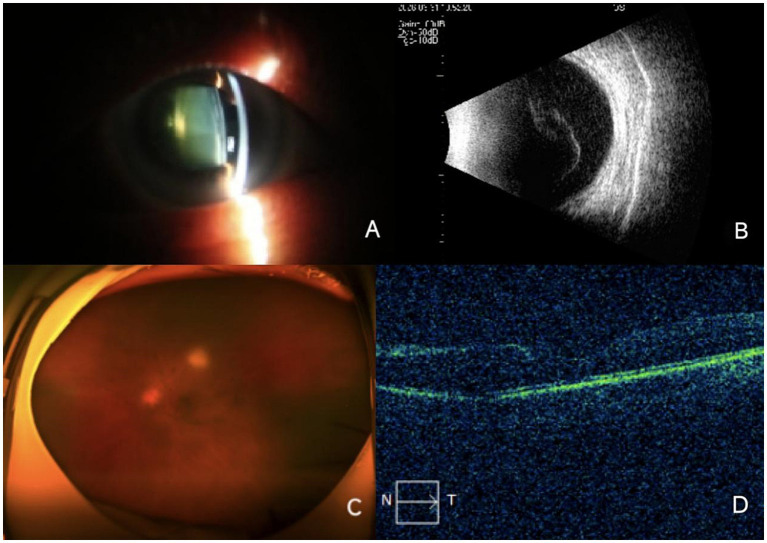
Pre-treatment images of the affected left eye. (A) Anterior segment photography showed marked lens opacity. (B) Pretreatment ocular B-scan ultrasonography revealed scattered vitreous opacities within the vitreous cavity. (C) Scanning laser ophthalmoscopy (SLO) showed inflammatory vitreous haze in the left eye, with an indistinct yellowish-white triangular lesion approximately 1 disc diameter in size at the superior vascular arcade; the retinal image quality was poor. (D) OCT showed an irregular retinal pigment epithelium layer in the macular region, macular edema, and poor signal quality.
